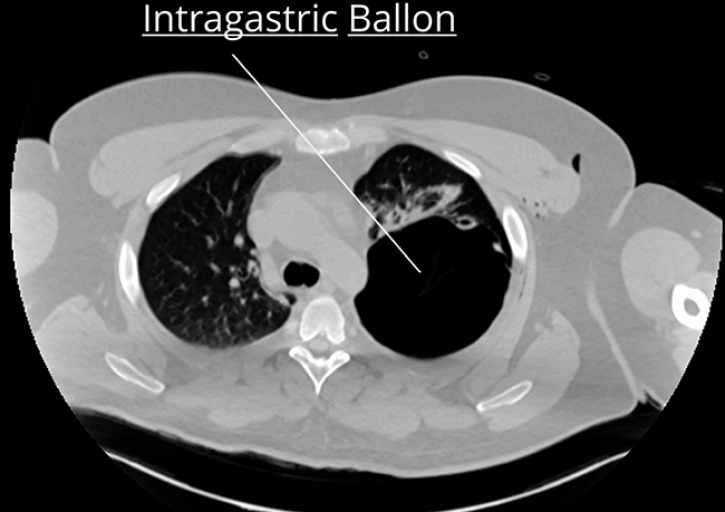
Preoperative computed tomography scan showing the intragastric balloon in the left pleural cavity.
